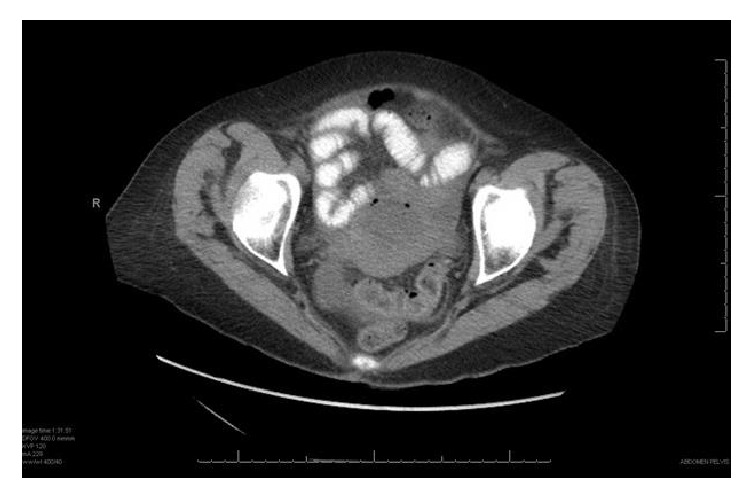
CT abdomen/pelvis showing pneumoperitoneum as well as air within the wall of the uterus.
